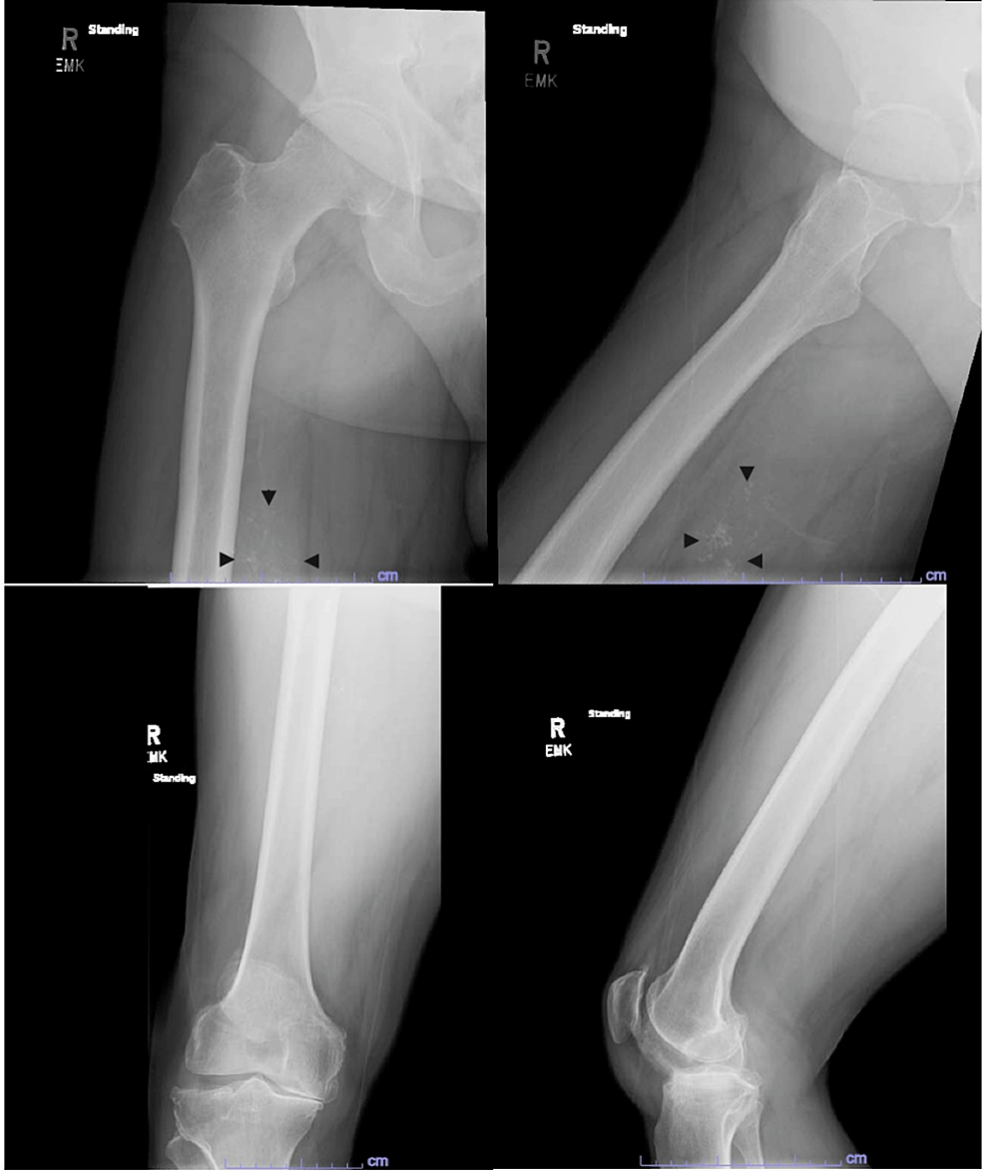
Multiple X-ray views of the femur demonstrating the calcification of a posterior soft tissue mass
